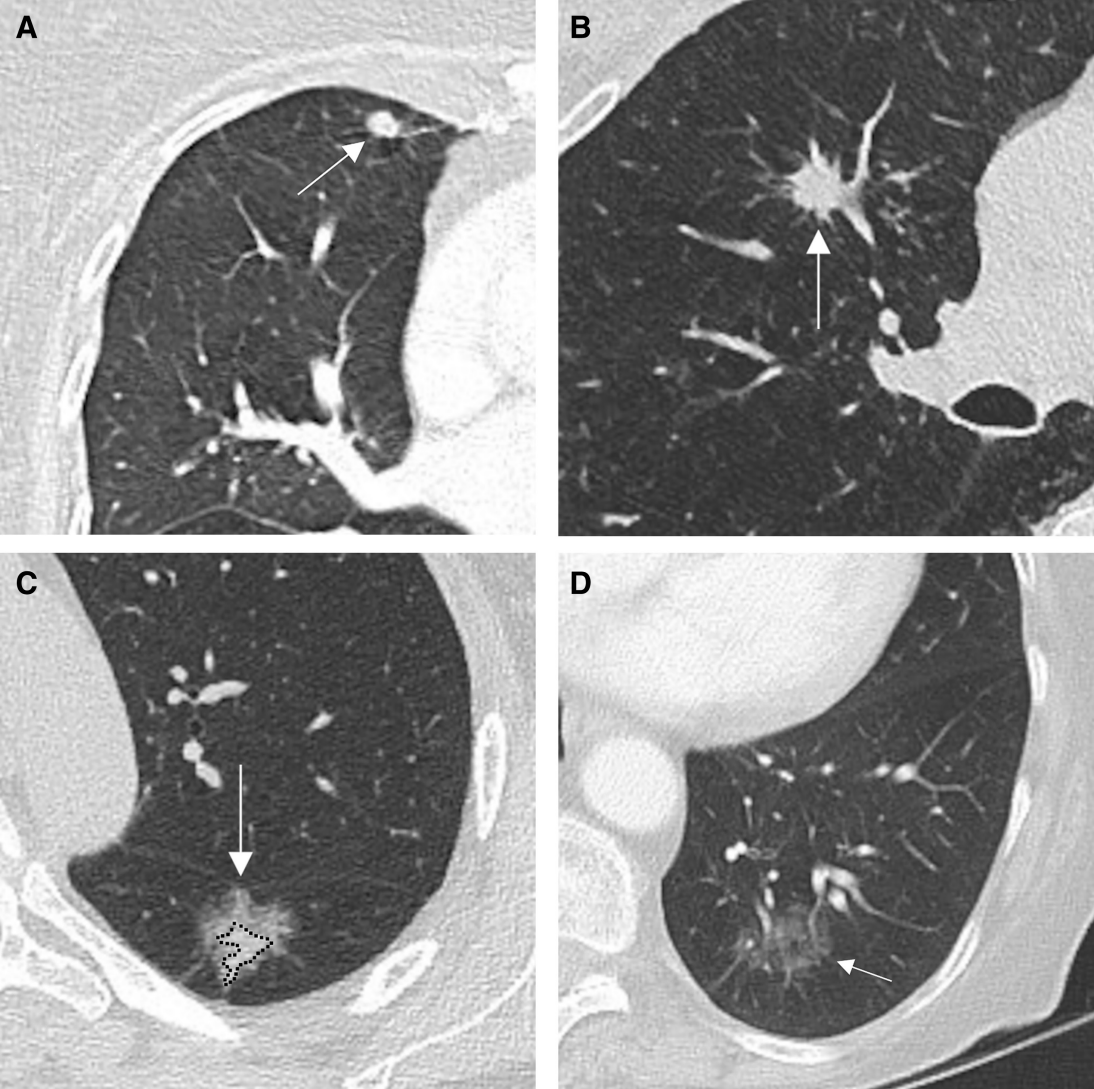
(A) Axial post‐contrast CT. Eight millimetre indeterminate pulmonary nodule in the right upper lobe (white arrow), solid with a smooth margin and subtle microlobulation. (B) Axial non‐contrast CT. Eleven millimetre solid nodule in the right upper lobe (white arrow). The spiculated margin increases the radiological likelihood of malignancy. (C) Axial non‐contrast CT. Twenty millimetre part solid nodule in the left lower lobe (white arrow). Both the ground glass and solid (dotted line) components have complex margins, limiting reproducibility of manual measurement and accuracy of automated volumetry. Direct visual comparison is critical to determine change over time. (D) Axial post‐contrast CT. Twenty‐five millimetre non‐solid nodule in the left lower lobe (white arrow). Blood vessels are visible through the lesion, hence non‐solid.
